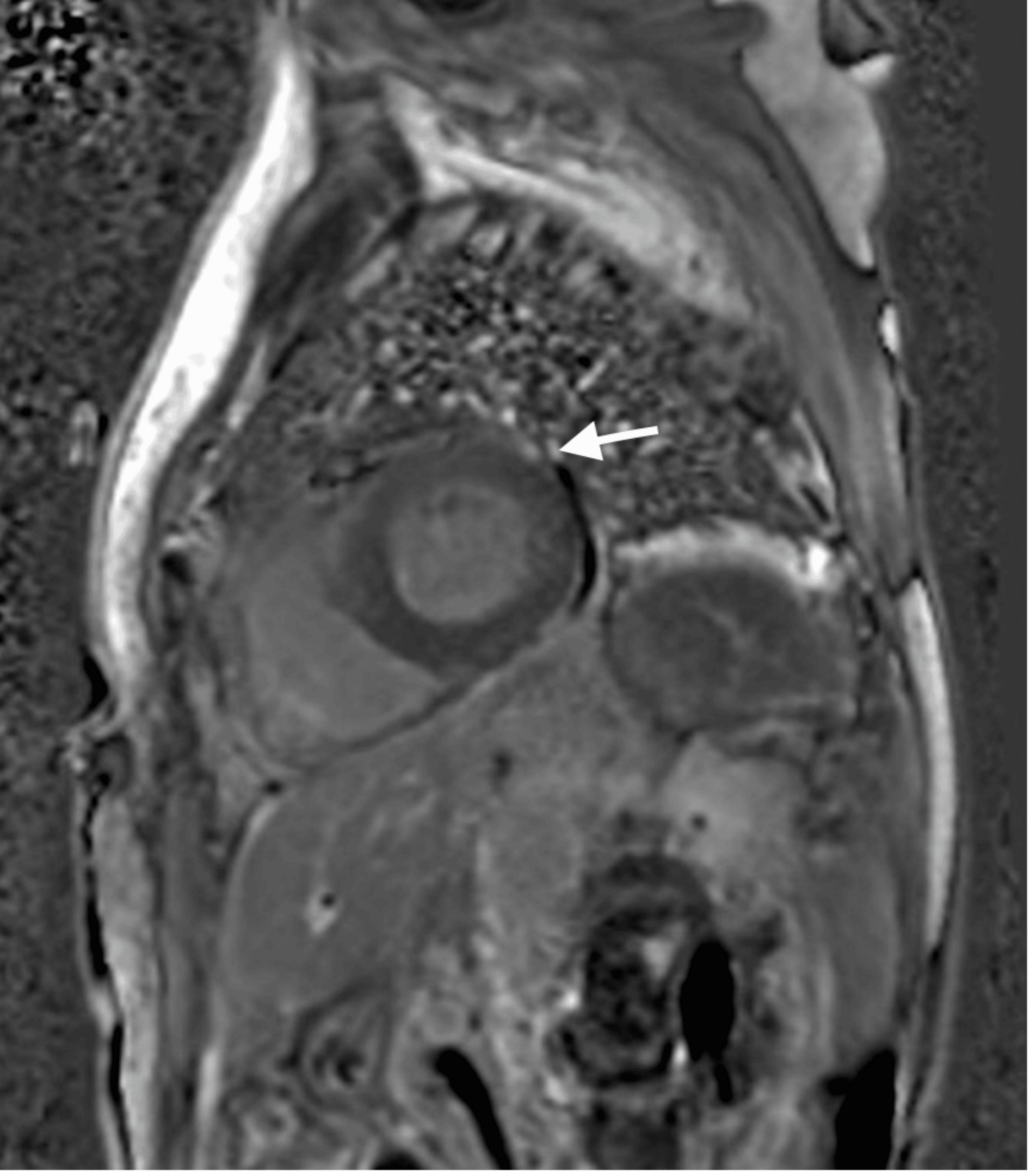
Late gadolinium enhancement cardiac MRI Cardiac MRI showing diffuse late gadolinium enhancement, predominantly in the basal and mid-cavity segments of the anterior and anteroseptal walls. These findings were less evident than usual as the patient had already been started on corticosteroids three days before. MRI: magnetic resonance imaging
